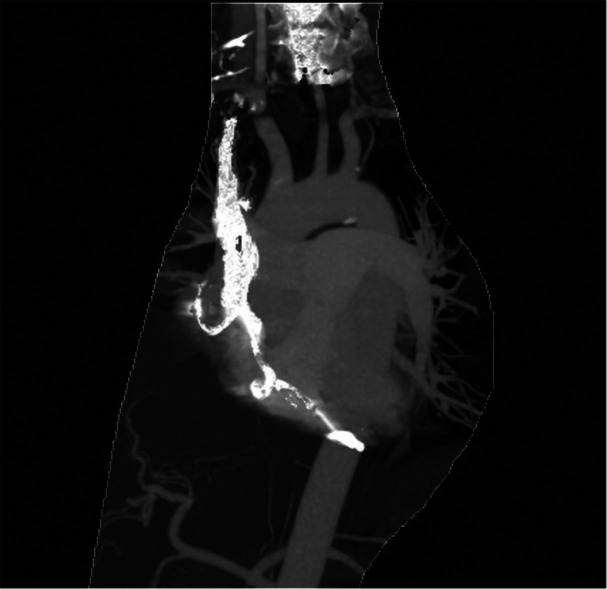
Chest CT scan demonstrating severe calcification surrounding the cardiac implantable electronic device leads. This imaging was performed to assess the extent of calcification and inform lead extraction planning, highlighting areas of significant calcified encasement.
